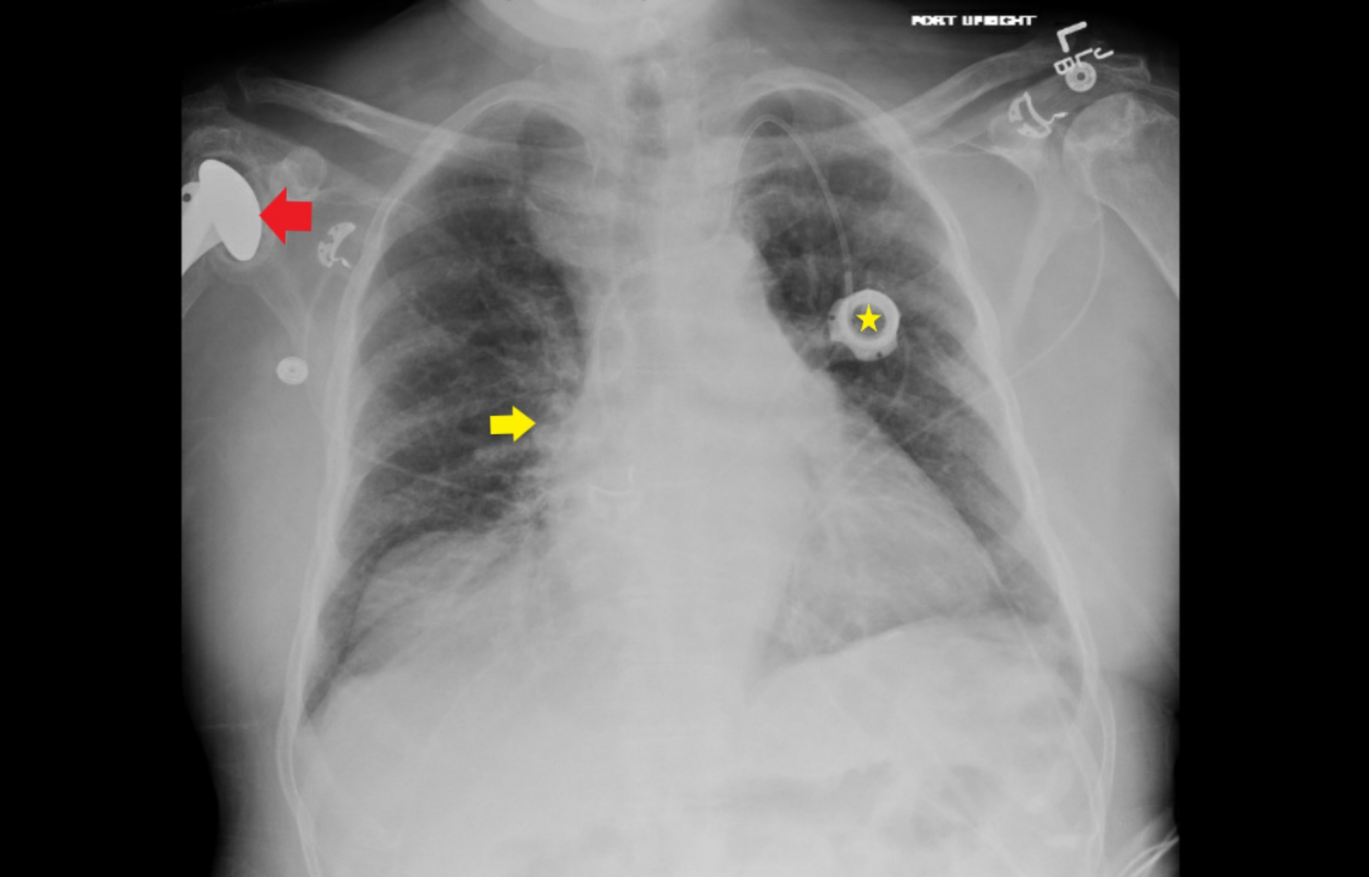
Chest X-ray: bilateral pulmonary hilar congestion (yellow arrow) and bilateral interstitial opacities; left chest wall port (yellow star) with catheter tip at the superior cavoatrial junction; left humeral head osteonecrosis with partially visualized right humeral prosthesis (red arrow)
